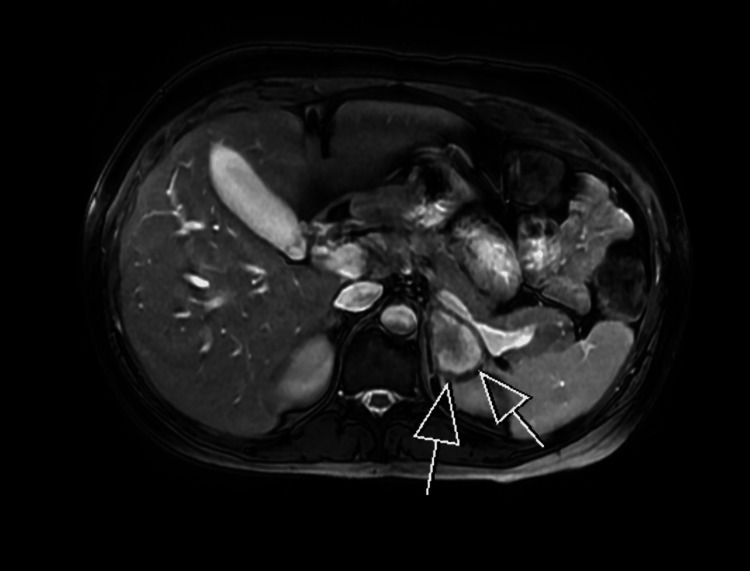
MRI adrenal showing an enlarged left adrenal gland.
